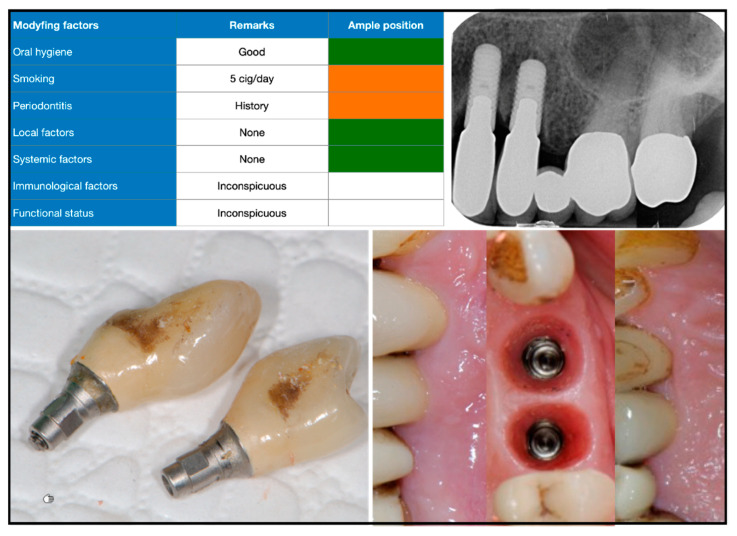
Initial findings in patient with history of periodontitis and smoking as co-factor (risk profile or traffic light, top left). Five years after implantation, recurrent abscesses formed at this site. X-ray image six years after operation shows vertical degradation patterns of peri-implant bone (top right).
